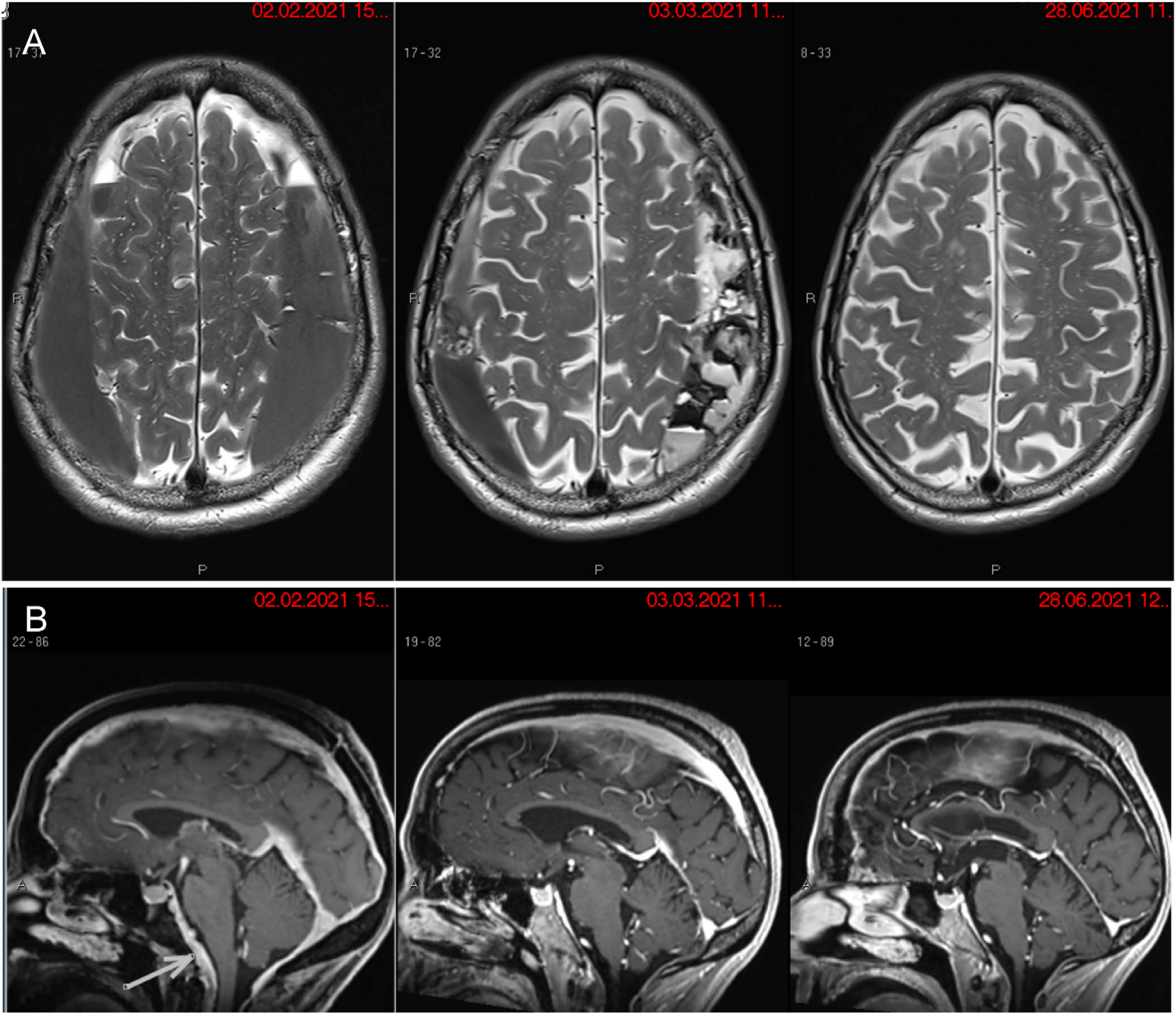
(A) Axial MRI images of the brain displaying the course of regression of a bilateral subdural hematoma in the fronto-parietal region, leading to a compression of both cerebral hemispheres without a midline shift. (Left) Six days preoperative. (Middle) Three weeks postoperative, a slight subsidence of fluid accumulation was observed with a normalization of the ventricular system and reappearance of fronto-parietal cerebral sulci. (Right) Five months postoperative with complete regression of the large subdural hematomas. (B) Sagittal MRI images of the brain showing regression of venous compartment enlargement due to a liquor-leak syndrome. (Left) Four days preoperative. (Middle) Three weeks postoperative. (Right) Five months postoperative.
